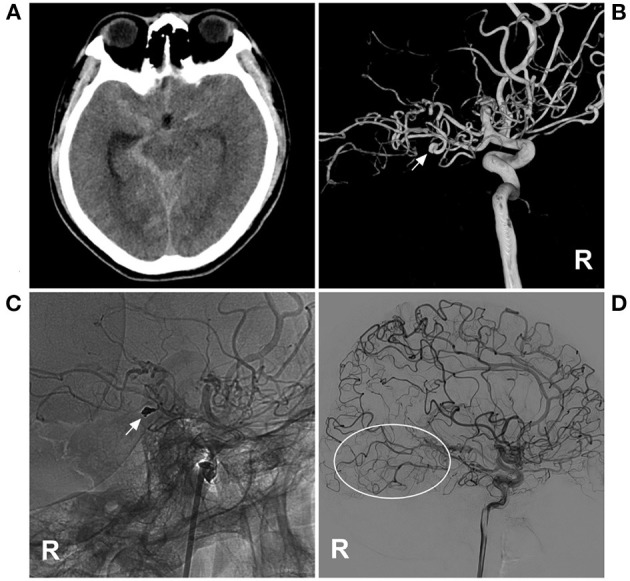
PAO of the PCA aneurysm in MMD in case 4 in Table 1. (A) CT showed subarachnoid hemorrhage focusing on the right ambient cistern. (B) Three-dimensional DSA of the right ICA showed an aneurysm (arrow) at the P2–3 segment of the PCA. (C) Unsubtracted DSA of the right ICA showed that the aneurysm and proximal parent artery underwent PAO by coiling (arrow). (D) Follow-up DSA of the right ICA showed no recurrence of the aneurysm, and the collateral circulation beyond the coils was abundant (circle). CT, computed tomography; DSA, digital subtracted angiography; ICA, internal carotid artery; MMD, moyamoya disease; PAO, parent artery occlusion; PCA, posterior cerebral artery; R, right.
